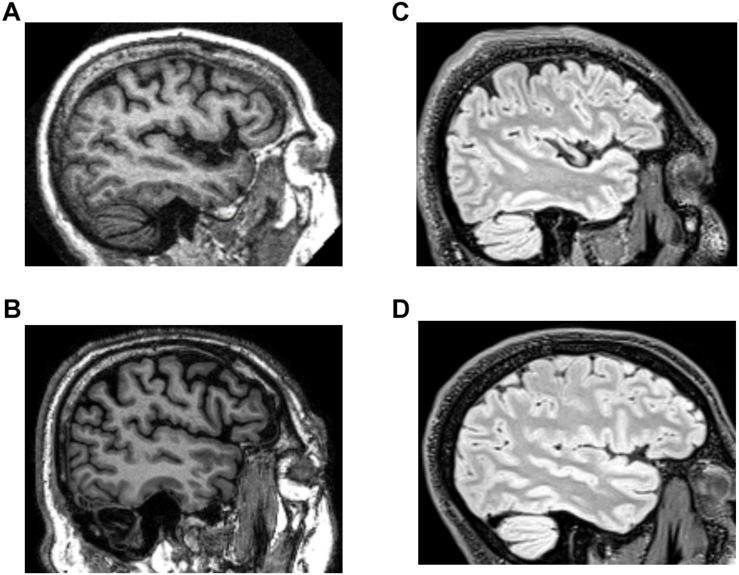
Example images from the structural brain MRI databases. (A,B) T1 images from the OASIS and ADNI databases. (C,D) T2-weighted FLAIR images from the TNS database (healthy subject, C; patient subject, D).
